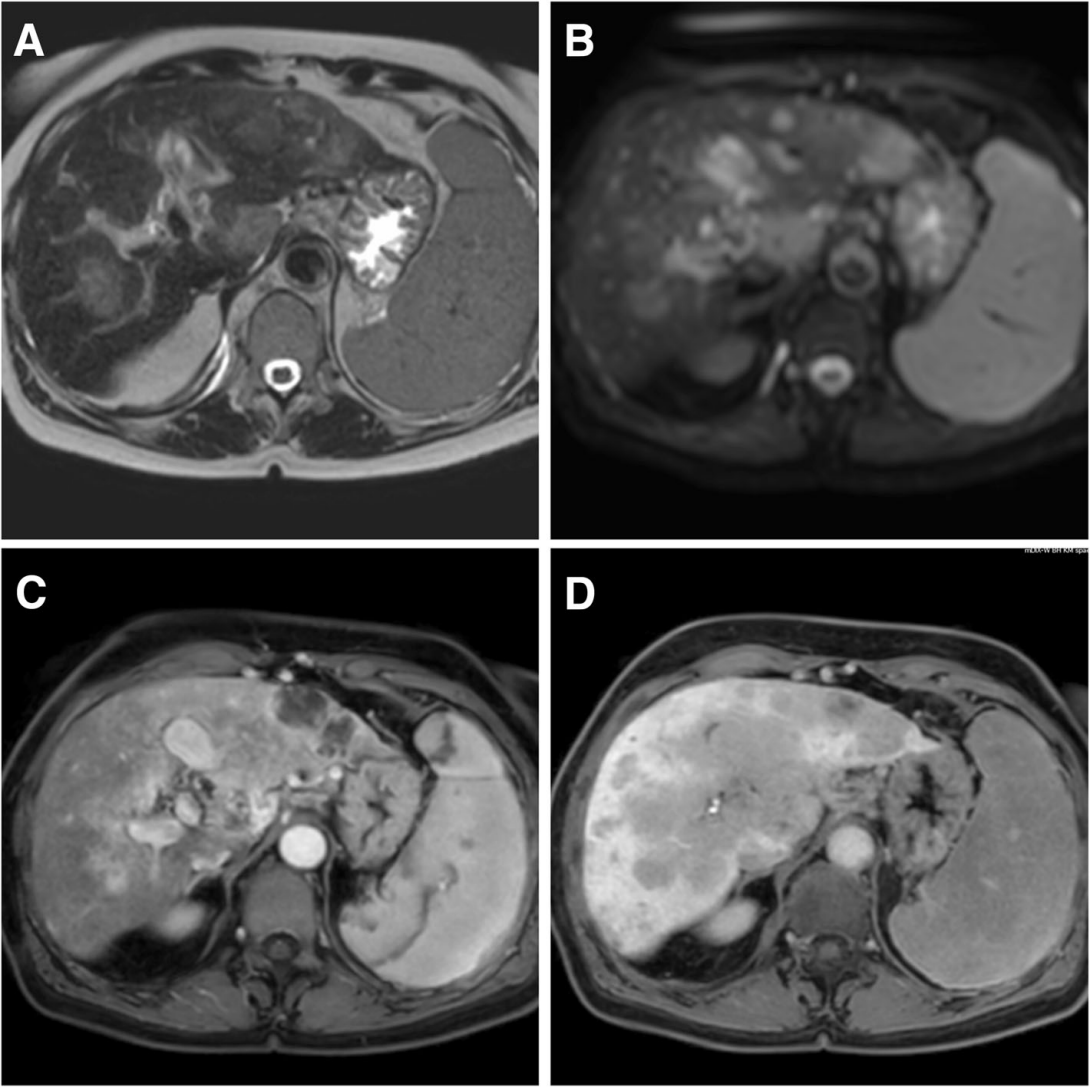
Epithelioid hemangioendothelioma of the liver in a 18-year-old woman. MR images depict diffuse spread of typical EHE tumor nodules over the entire liver. T2w (a), diffusion-weighted imaging (b), Dixon fat-water imaging in the early (c), and late phase (d) after administration of gadoxetic acid. Due to accompanying liver cirrhosis (child B), the patient rapidly developed progressive liver failure and could not undergo surgery or systemic therapy
